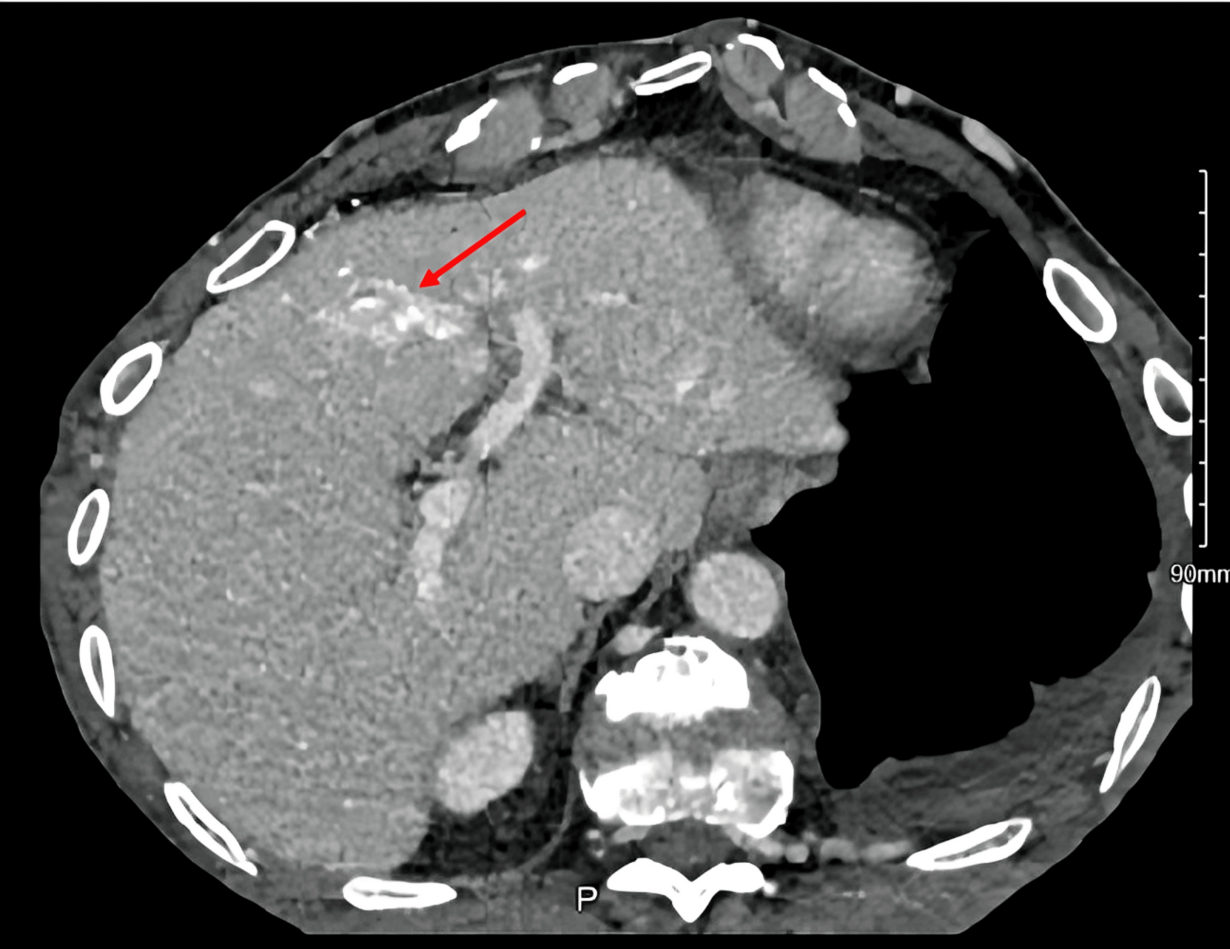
Axial view of CT abdomen showing focal area of contrast accumulation in segment IV of liver (arrows) showing hot quadrant sign.
